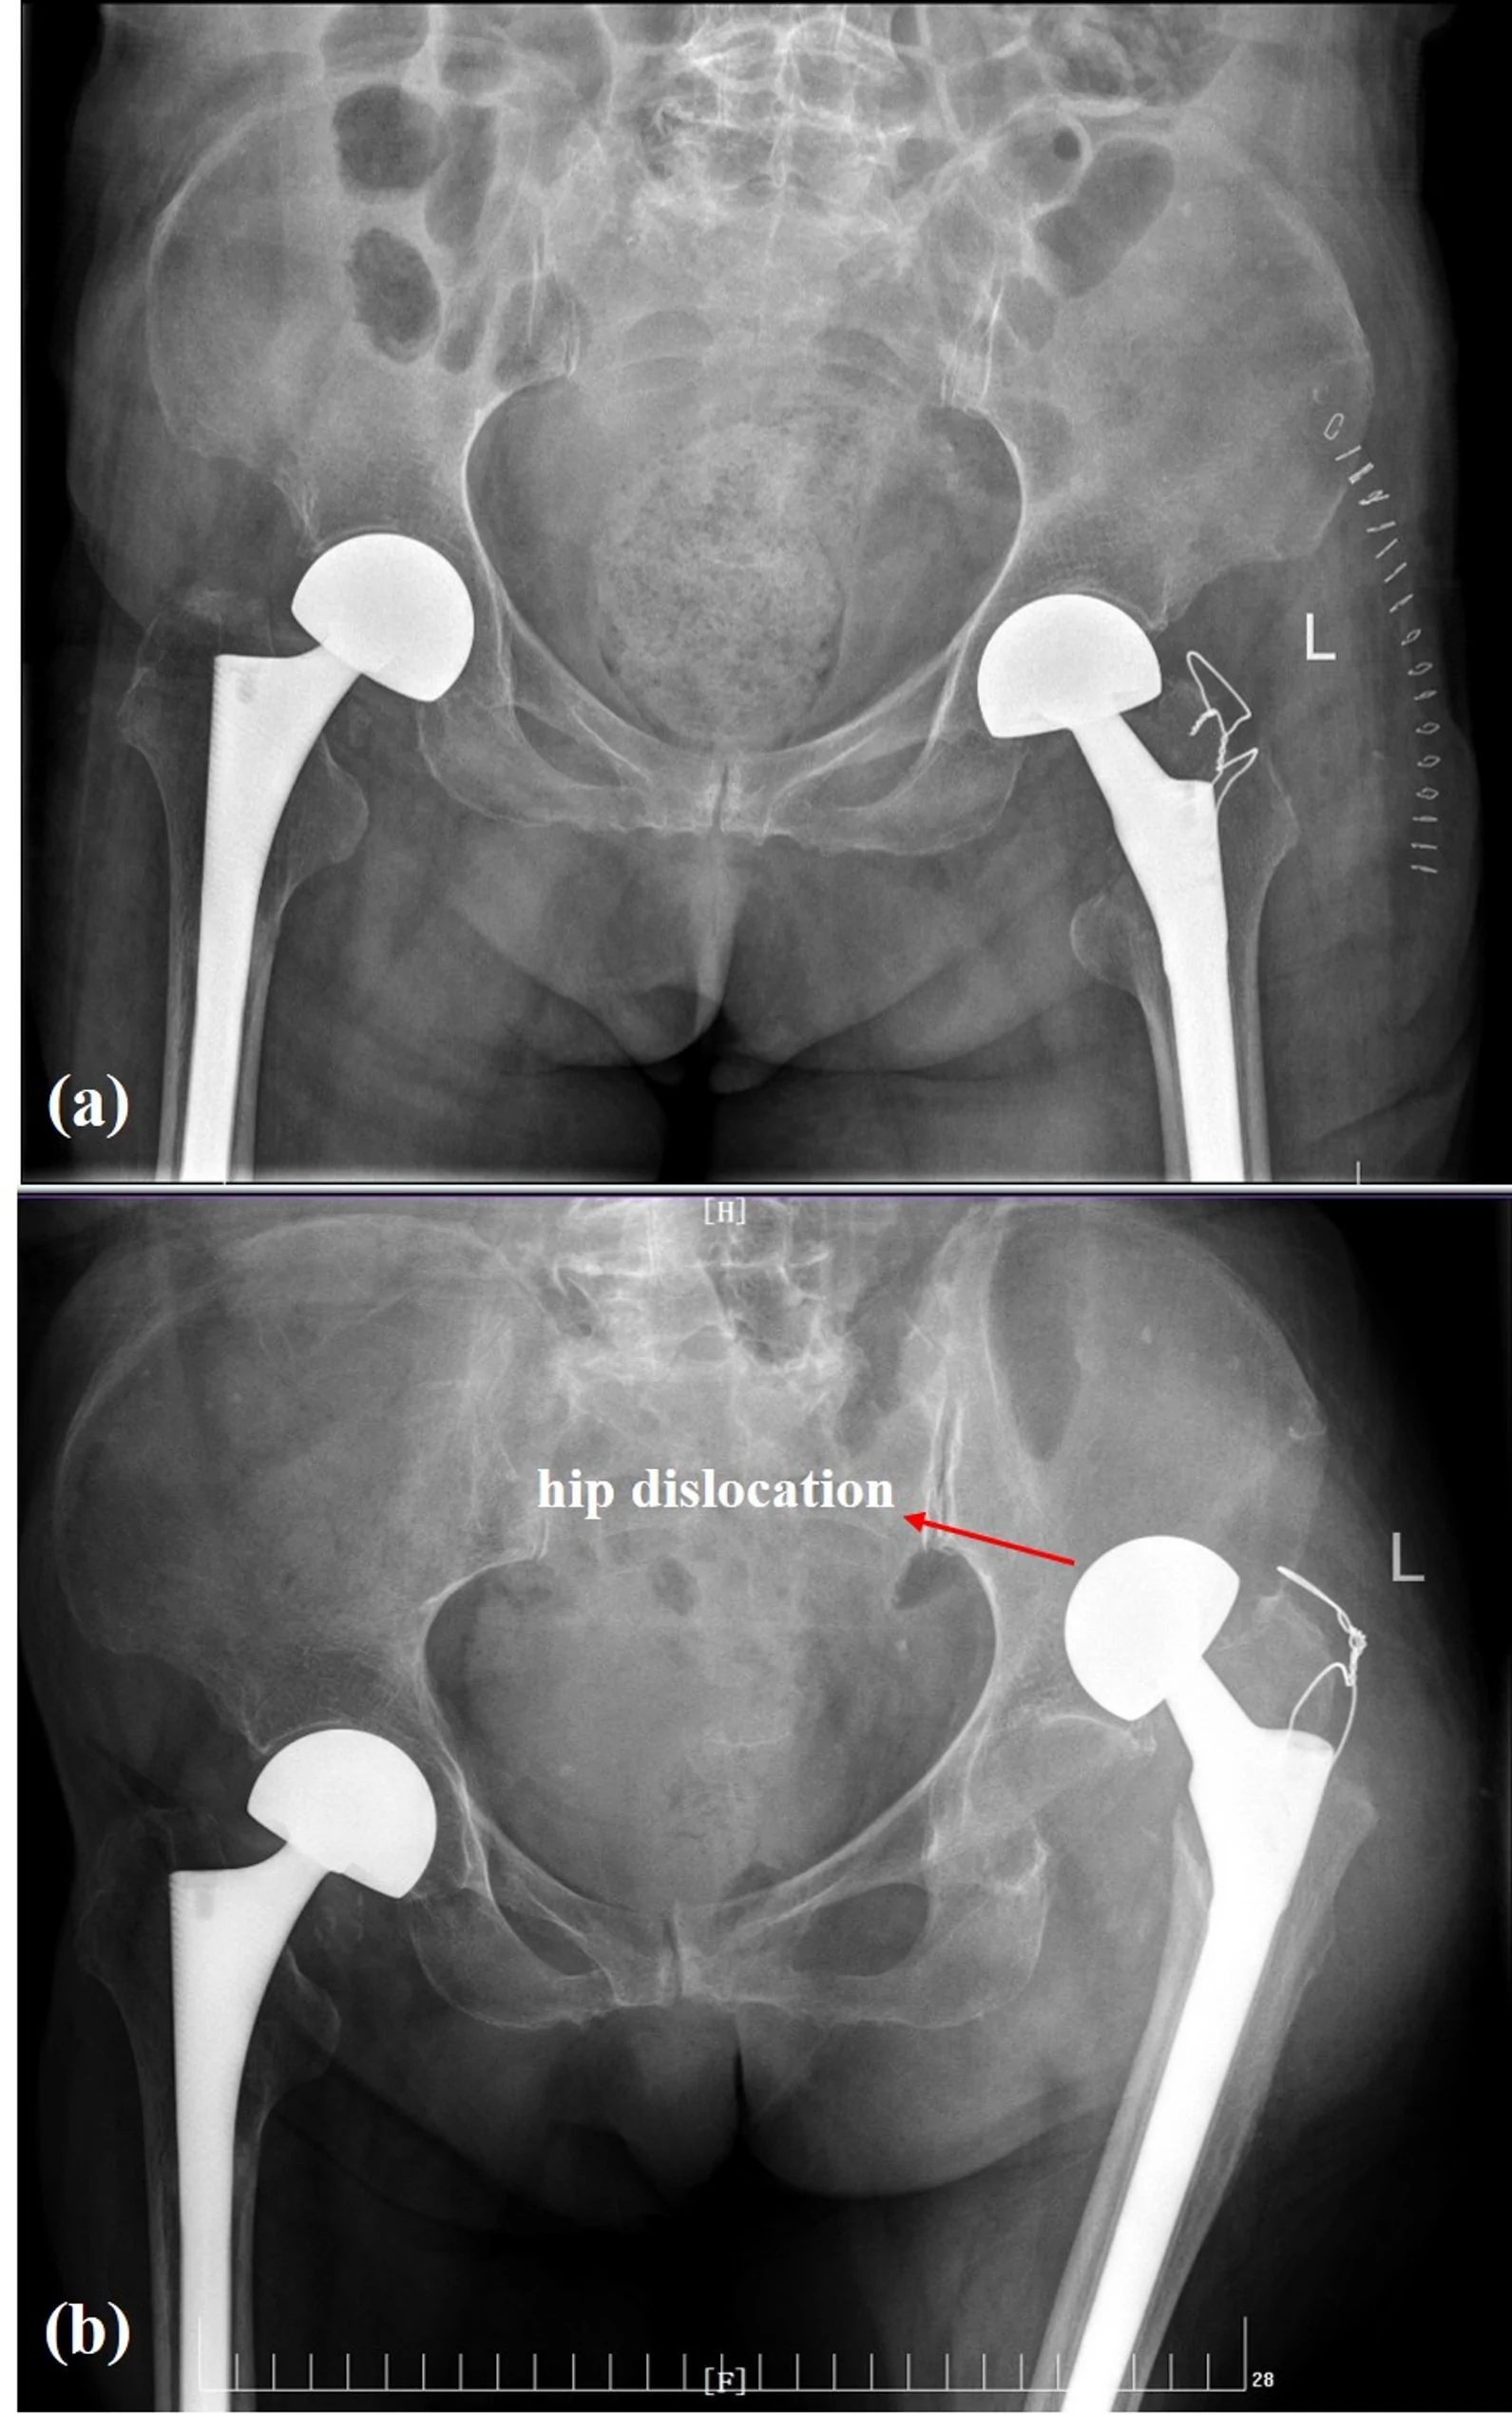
Radiographs of a female patient aged 60–70 years with left femoral intertrochanteric fracture treated with hemiarthroplasty. a Postoperative radiograph at discharge: no obvious periprosthetic abnormality. b Radiograph obtained 2 years postoperatively: hip dislocation
